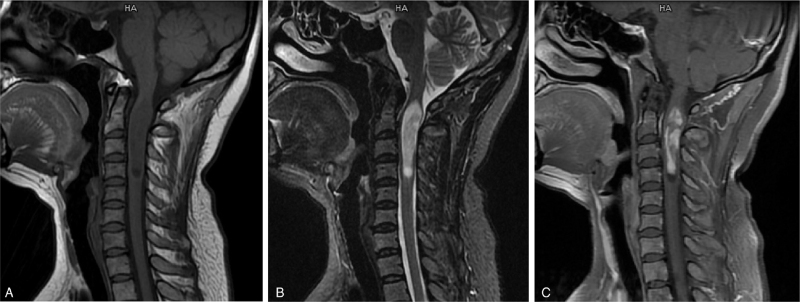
Magnetic resonance imaging reveals the primary intramedullary lesion extending from C1 to C4, characterized by T1-weighted imaging hypointensity (A) and T2-weighted imaging hyperintensity (B), and intensity is enhanced after contrast medium administration (C).
